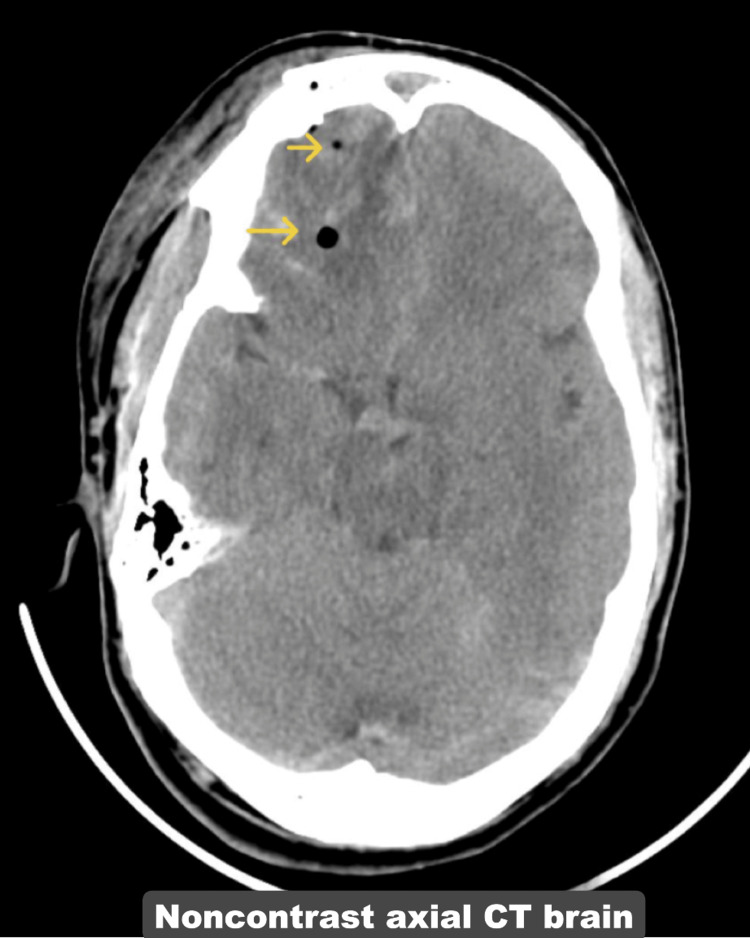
Day 0 initial non-contrast axial CT brain showing frontal contusions with air foci Yellow arrows show intracranial air foci (pneumocephalus) with surrounding hemorrhagic contusions in the right frontal region.
